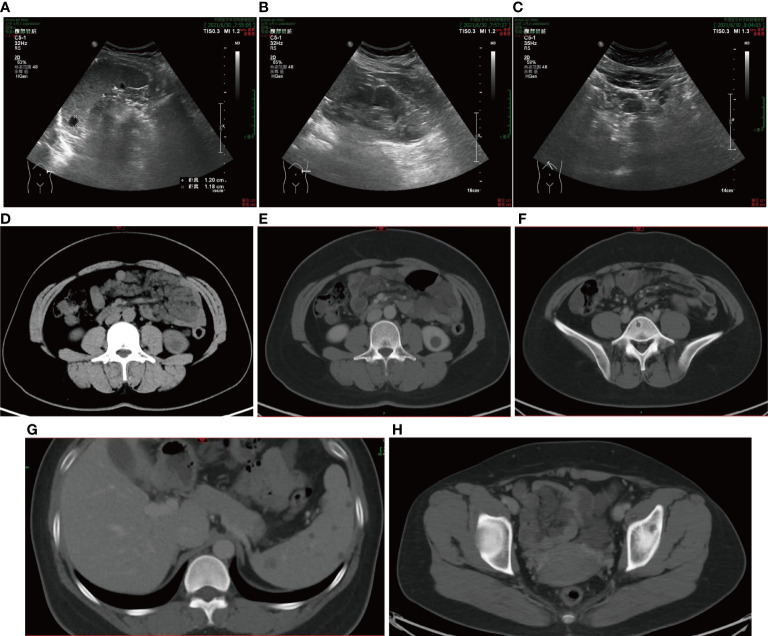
(A–C) Abdominal ultrasound observed multiple hypoechoic nodules in the spleen and the lower poles of the left kidney, and enlarged lymph nodes in the retroperitoneum. (D) Abdominal CT scan observed multiple diffuse low-density shadowing zones in the mesenteric area, which presented a doughnut shape. (E–H) Low-density shadowing zones can be observed in the left kidney, spleen, ilium, and the fifth lumbar vertebra in abdominal CT scan images.
